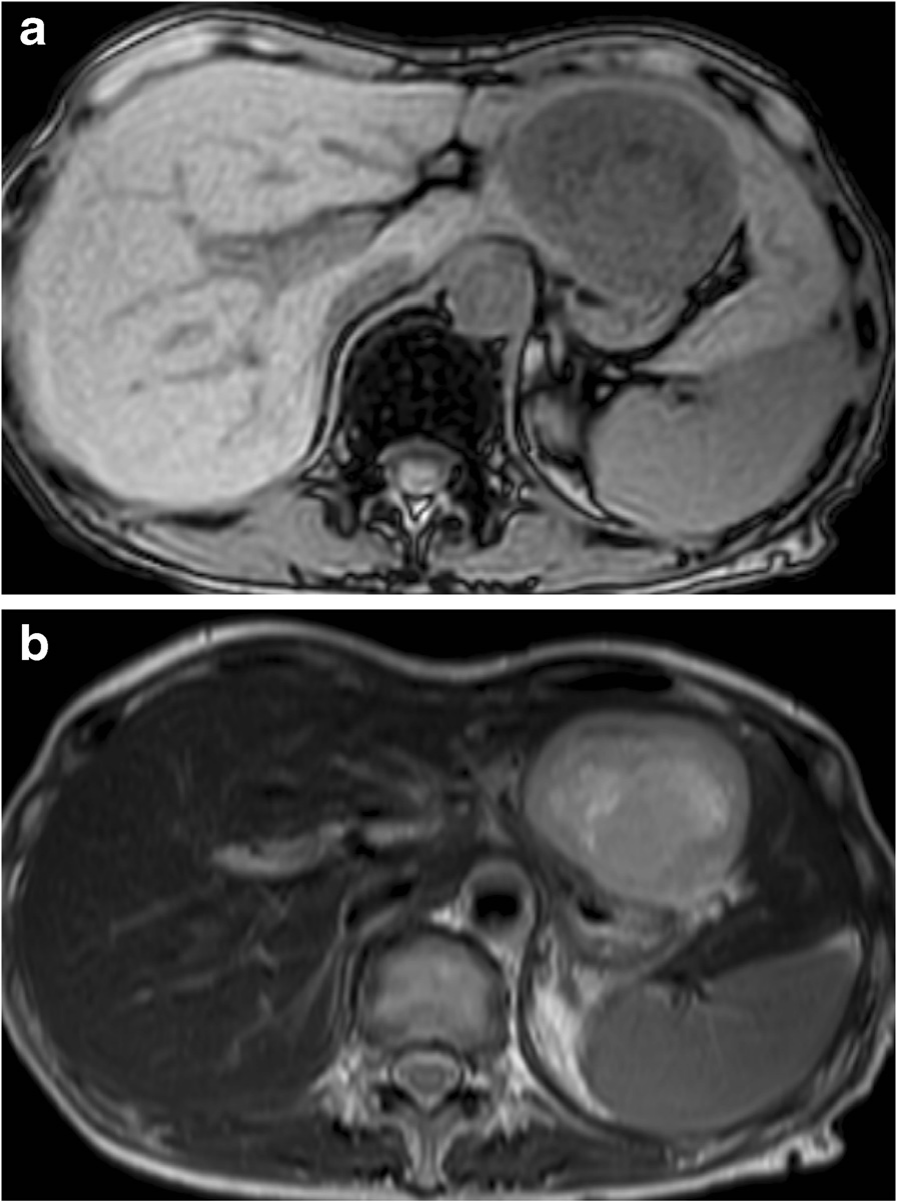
MRI scan showing a low- and high-intensity tumor on T1-weighted phase images (a) and T2-weighted phase images (b), respectively
